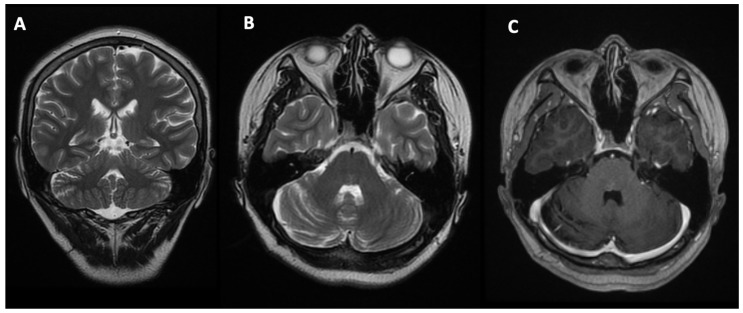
Follow-up MRI at 12 months after surgery. Coronal T2-weighted imaging (A), axial T2-weighted imaging (B), and post-contrast 3D T1-weighted imaging (3D AX BRAVO) (C) demonstrated stable postoperative findings with no evidence of tumour recurrence. The posterior fossa structures and cerebrospinal fluid pathways were preserved, with no signs of hydrocephalus or mass effect.
